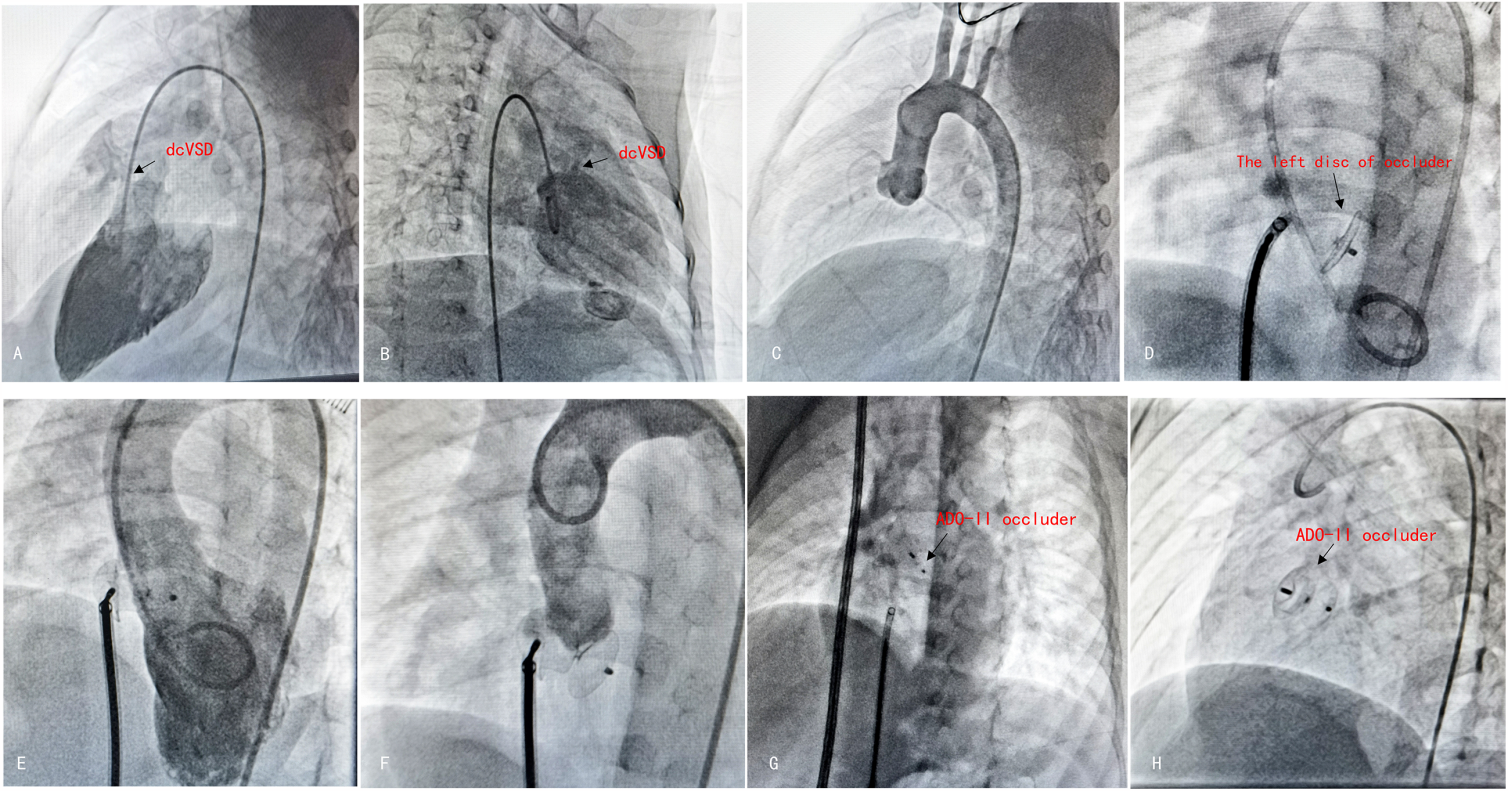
The procedure of antegrade dcVSD closure. (A,B) Left ventricular angiography was performed to confirm the position and size of dcVSD from two different angles—left anterior oblique at 60°cranial 20° (A) and right anterior oblique at 20° (B). (C) Ascending aortogram was performed to observe for AR and AVP. (D) After establishing the delivery pathway, the left disc was deployed at the appropriate position. (E,F) Left ventricular and aortic angiography were repeated to confirm the absence of RS and no exacerbation of AR. (G) Released the right disc. (H) A final radiograph was taken to confirm the occluder's position.
